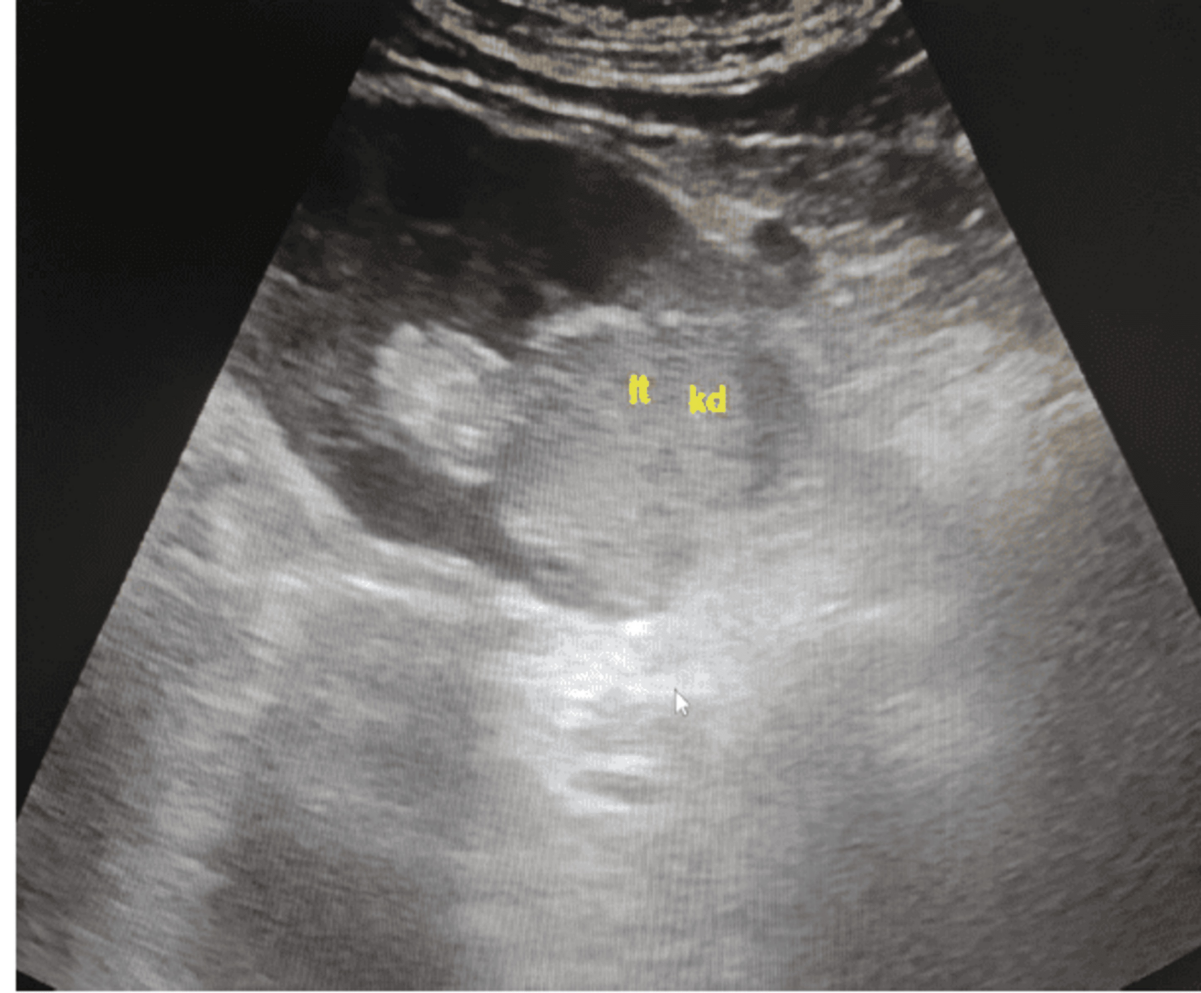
Ultrasound image of the left kidney demonstrating a heterogeneous hypoechoic mass involving approximately the lower one-third of the renal parenchyma. This finding raised suspicion of a renal tumor and prompted further imaging.
